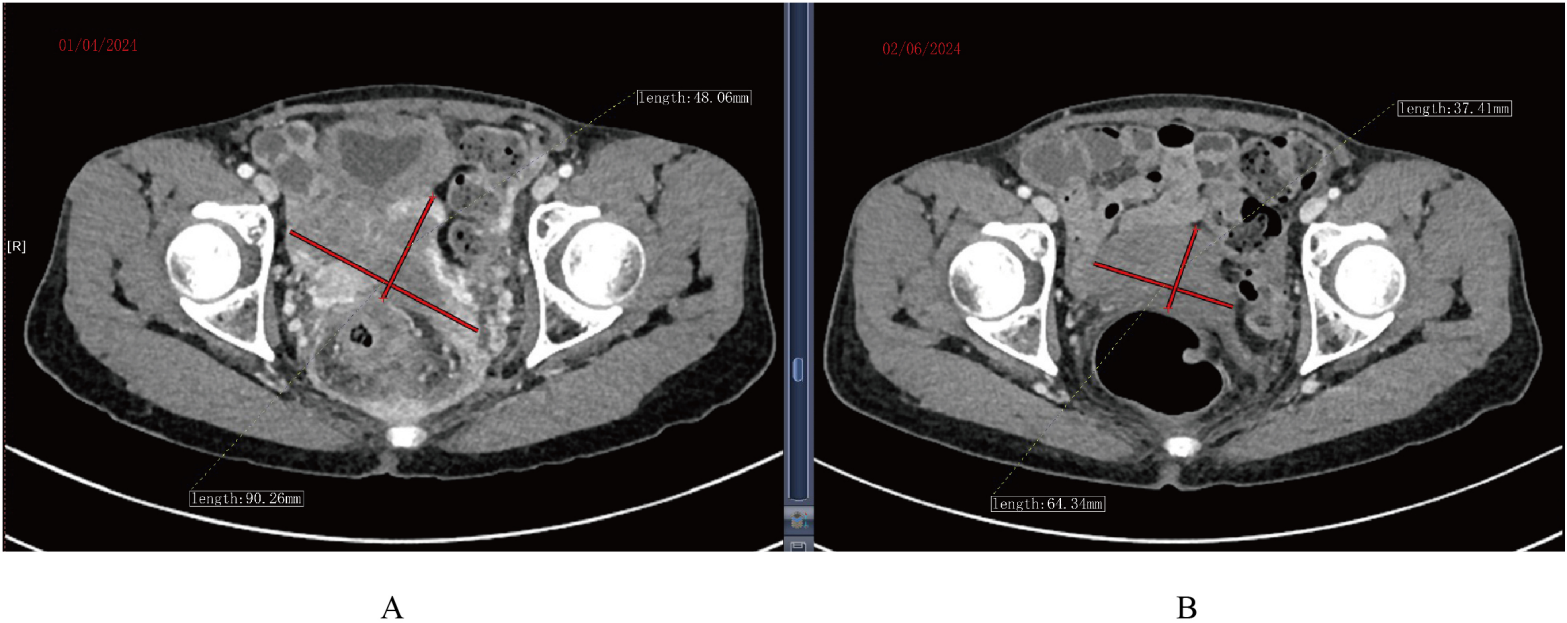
CT scan of tumor mass changes showing partial response 1 month post CAR-T cell infusion. (A) CT scan before infusion of CAR-T infusion. (B) CT scan 1 month post CAR-T cell infusion.
